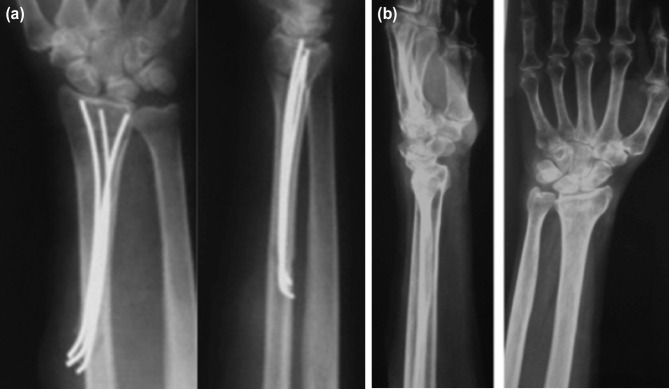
(a) Radiographic images showing union at the final follow-up with wires in-situ, and (b) following removal of wires.
